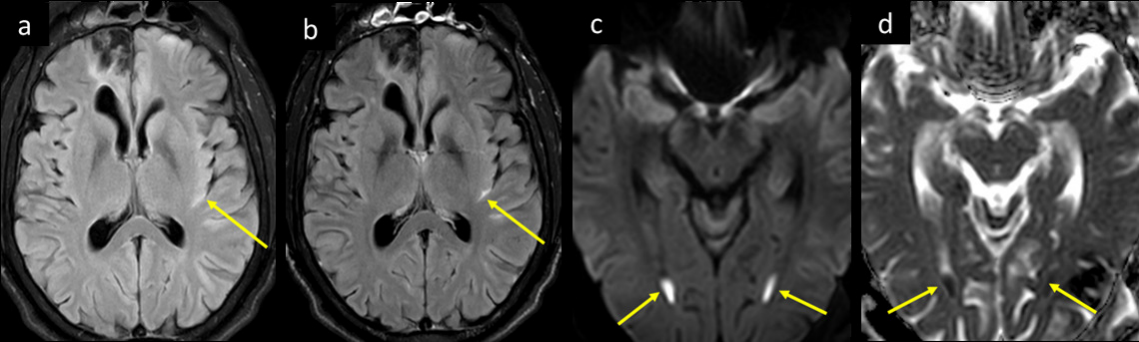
A 27-year-male patient with known post-traumatic seizures on antiepileptic mediations presented to ED with status epilepticus. He was found to be febrile and desaturated, the seizures were aborted, and he was intubated, and tested positive for COVID-19. Plain CT head (not shown) showed right anterior frontal old trauma changes and was otherwise unremarkable. His CSF analysis proved positive for Gram positive *Streptococcus pneumonia*. MRI head axial images at mid-ventricular level (a) *T*_2_-FLAIR before and (b) after i.v. contrast shows bright signal intensity in sylvian fissure, with post-contrast enhancement (long arrows in a and b), consistent with meningitis. Note the post-traumatic right anterior frontal encephalomalacia and gliotic changes (short arrow in a and b). Axial images at lower ventricular level (c) DWI b 1000 and (d) ADC map shows dependent pus with diffusion restriction within the occipital horns of the lateral ventricles bilaterally, representing ventriculitis. ADC, apparent diffusion coefficient; CSF, cerebrospinal fluid; DWI, diffusion-weighted image; ED,emergency department; FLAIR, fluid attenuated inversion recovery.
